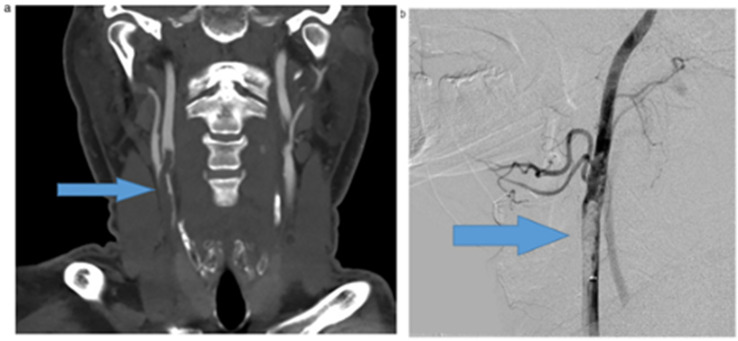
A significant amount of thrombus in the carotid artery. A man in his 50s who went to the doctor complaining of weakness in his left wrist was found to have positive serology for COVID-19. A significant subocclusive thrombosis of the right common carotid artery that extended into the internal and external carotid arteries was seen on the head and neck (a) CT angiography (arrows). The CT perfusion analysis revealed an acute infarct in the right superior frontal lobe as well as a wide area of elevated Tmax in the right cerebral hemisphere which involved both the right frontal and parietal lobes, indicating an area that may be at risk for additional infarction (box). (b) Immediately afterwards, endovascular chemical thrombolysis of the right carotid artery was carried out [144].
